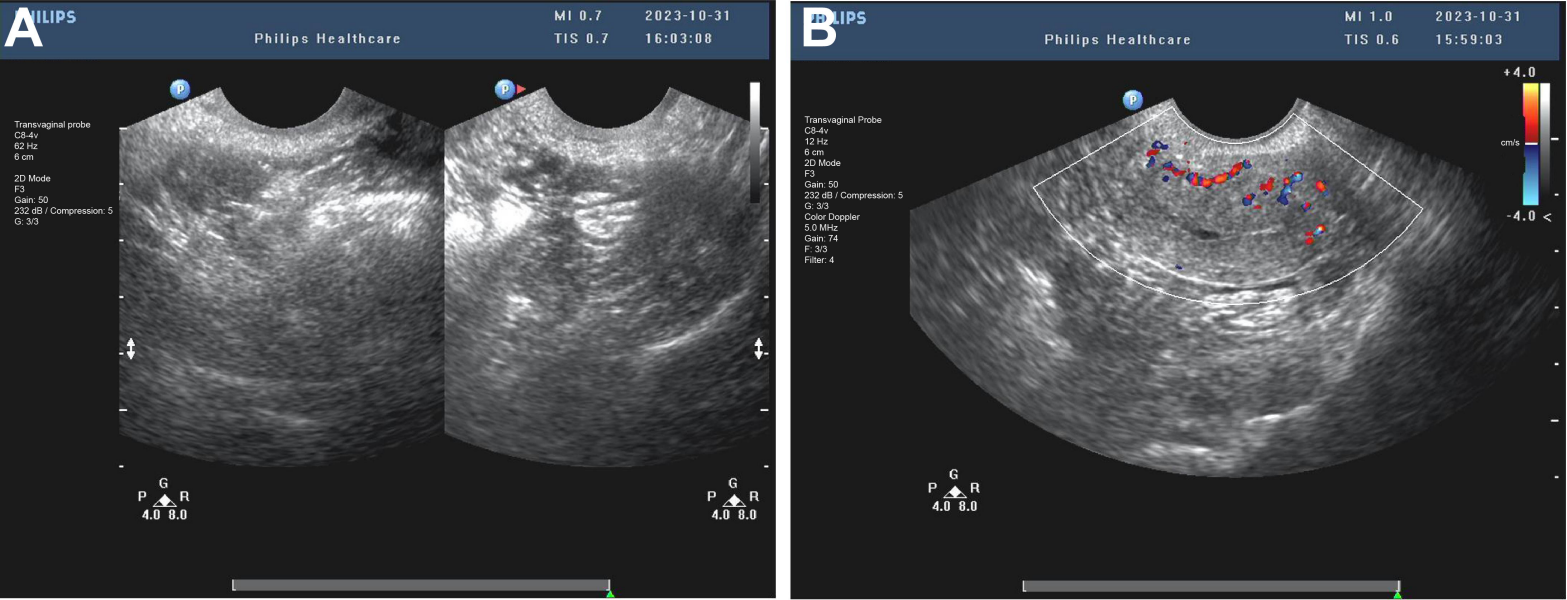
Postoperative transvaginal ultrasound assessment of the uterus and adnexa. (A) The postoperative ultrasound image shows a retroverted uterus of normal size, with a length of 36 mm, an anteroposterior diameter of 25 mm, and a transverse diameter of 37 mm. (B) The postoperative ultrasound shows no abnormal masses in the bilateral adnexa. The left ovary measures 18 mm × 11 mm, and the right ovary measures 20 mm × 11 mm, indicating that the surgery did not cause any ovarian or adnexal lesions.
